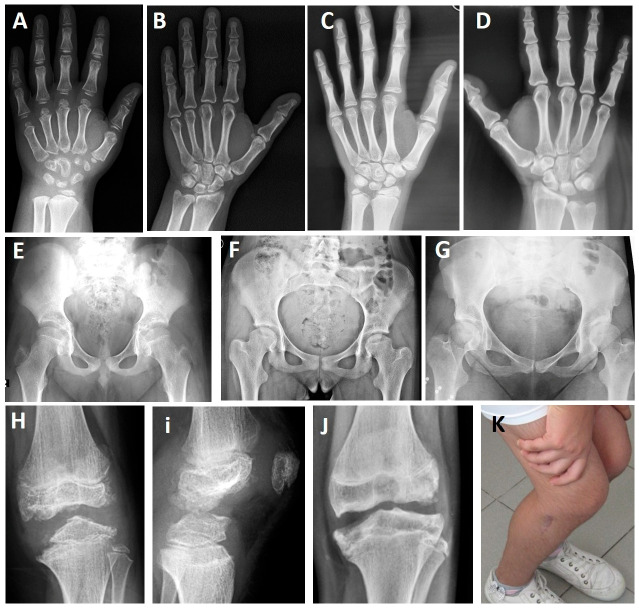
Patients with MED2 and MED3. P11 at age 10 years (A,E,H,I) and P12 at age 45 years (B) with MED2. P13 at age 14 years (C,F,J) and P14 at age 49 years (D,G,K) with MED3. Small and irregular carpal bones are seen in childhood (A,C), while slight irregularities of the carpal bones are observed in adulthood (B,D) on hand radiographs. Note the normal features (E) in the patient with MED2 on the pelvic radiograph, while a flat femoral head (F) and a small femoral head with a short femoral neck are seen in MED3 (G). Irregular knee epiphyses are observed in patients with MED2 and MED3 (H–J). Knee limitation observed in P13 (K).
